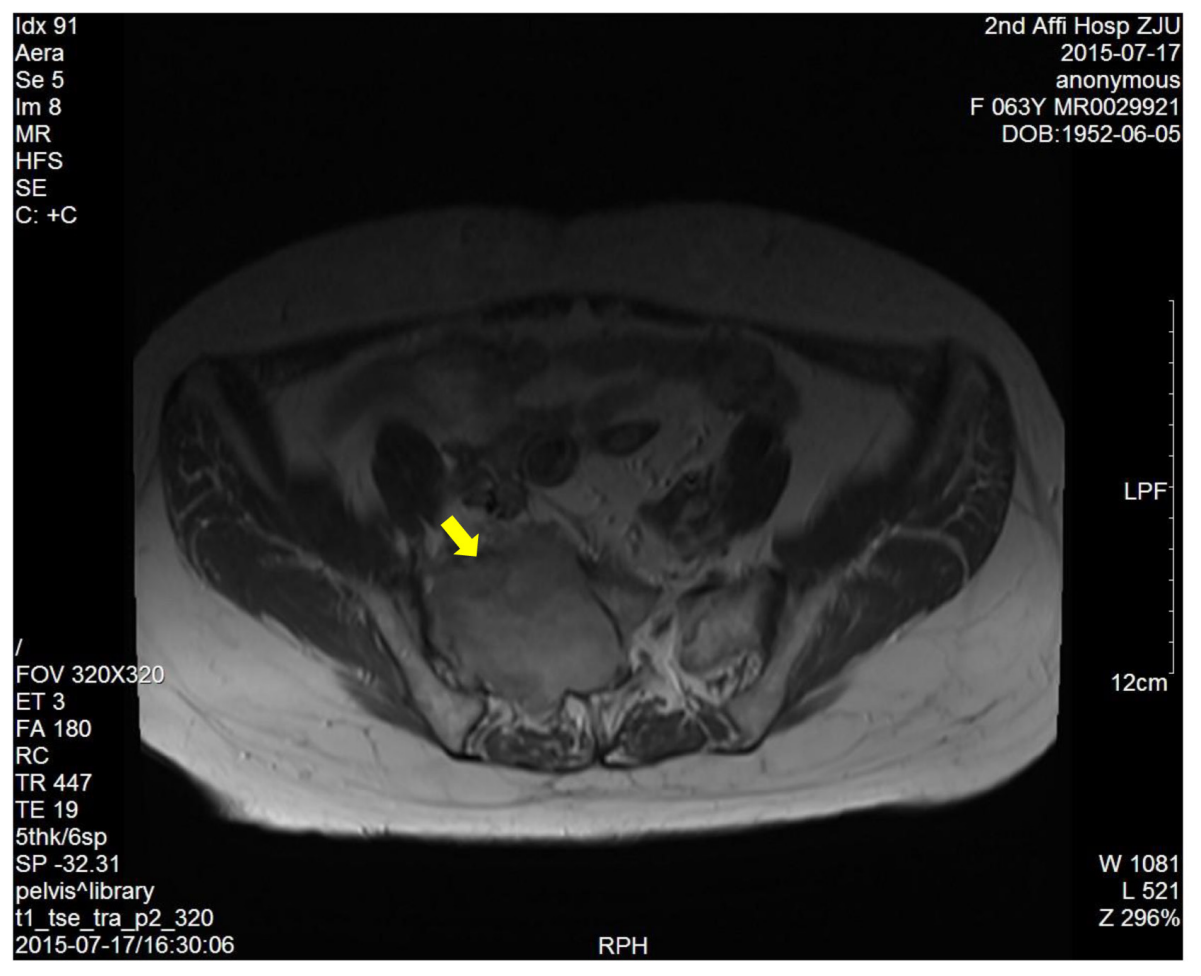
The enhanced T1-weighted image showed a right sacral mass with uneven enhancement
